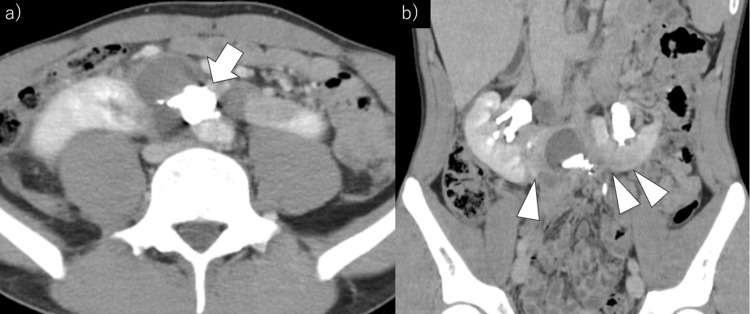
Contrast-enhanced computed tomography was performed one month after the procedure. (a) Axial view: Good deposition of N-butyl-2-cyanoacrylate and lipiodol (arrow) and reduction of the retroperitoneal hematoma are observed. (b) Coronal view: Poor contrast enhancement in the renal isthmus and both renal lower poles (arrowheads) is observed, but no extension of the renal infarction is noted.
